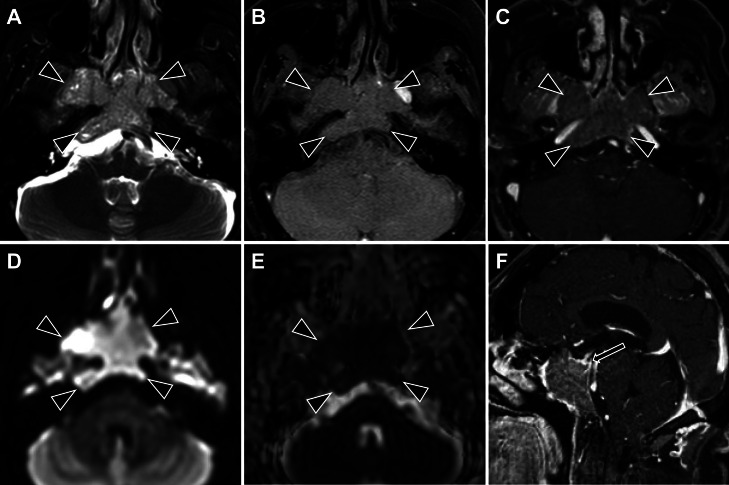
The mass in the sphenoid sinus on MRI. Axial images include a T2-weighted image (A), noncontrast T1-weighted image (B), postcontrast T1-weighted image (C), a diffusion-weighted image (D, b = 1000 s/mm²), and an ADC map (E). A sagittal postcontrast T1-weighted image is also shown (F). On imaging, the tumor demonstrates heterogeneous signal intensity on T2WI, composed of isointense and hyperintense signals relative to the skeletal muscles (A). The lesion is isointense on precontrast T1WI (B) and reveals weak, heterogeneous enhancement following gadolinium administration (C). Diffusion-weighted imaging reveals restricted diffusion, evidenced by high signal intensity (D) and low ADC values (mean: 0.796, min: 0.601 × 10⁻³ mm²/s). There is no evidence of intratumoral necrosis or cystic components. The tumor shows extensive invasion, extending into the right infratemporal fossa, destroying the clivus and dorsum sellae, and infiltrating the sella turcica and cavernous sinus (C, F). This results in superior displacement of the pituitary gland (F, arrow). The internal carotid artery remains patent without stenosis (C). Perineural invasion is not observed.
